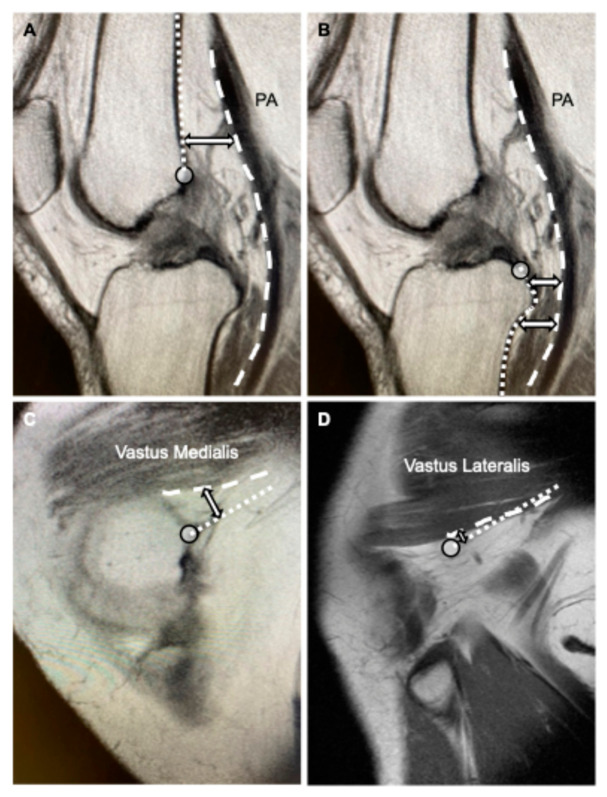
Measurement methods defined on open magnetic resonance imaging (MRI) images. (A) Measurement of the vascular safety margin on the femoral side (F-PA): On the sagittal multi-planar reconstruction (MPR) image, the distance from the posterior cortex of the femur (dotted line) to the anterior wall of the popliteal artery (PA) (dashed line) was measured perpendicular to the longitudinal axis of the femur. The circle marker indicates the zero reference point (0 cm) at the intersection of the intercondylar line (Blumensaat’s line) and the posterior femoral cortex. (B) Measurement of the vascular safety margin on the tibial side (T-PA): On the sagittal MPR image, the distance from the posterior cortex of the tibia (dotted line) to the anterior wall of the PA (dashed line) was measured perpendicular to the longitudinal axis of the tibia. The circle marker indicates the zero reference point (0 cm) at the intersection of the tibial plateau (joint line) and the posterior tibial cortex. (C) Measurement of surgical exposure for the vastus medialis (F-VM): On the medial sagittal MPR image, the distance from the posteromedial femoral cortex (dotted line) to the posterior border of the vastus medialis (F-VM) (dashed line) was measured perpendicular to the longitudinal axis of the femur to evaluate the potential space available for muscle retraction. The circle marker indicates the zero reference point (0 cm) at the intersection of the intercondylar line and the posteromedial femoral cortex. (D) Measurement of surgical exposure for the vastus lateralis (F-VL): On the lateral sagittal MPR image, the distance from the posterolateral femoral cortex (dotted line) to the posterior border of the vastus lateralis (F-VL) (dashed line) was measured similarly. The circle marker indicates the zero reference point (0 cm) at the intersection of the intercondylar line and the posterolateral femoral cortex. The double-headed arrows in each panel indicate the measurement direction, perpendicular to the longitudinal axis of the respective bone.
